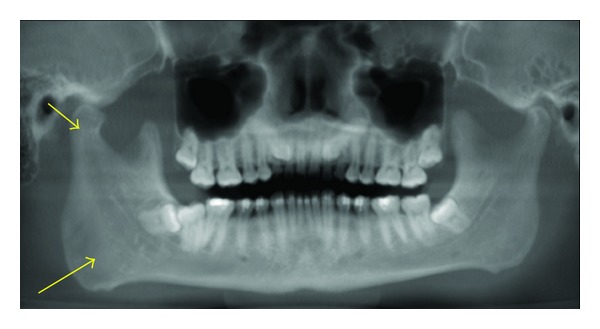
Hemihyperplasia seen as a much larger right side of the mandible on this panoramic reconstruction CBCT image.
